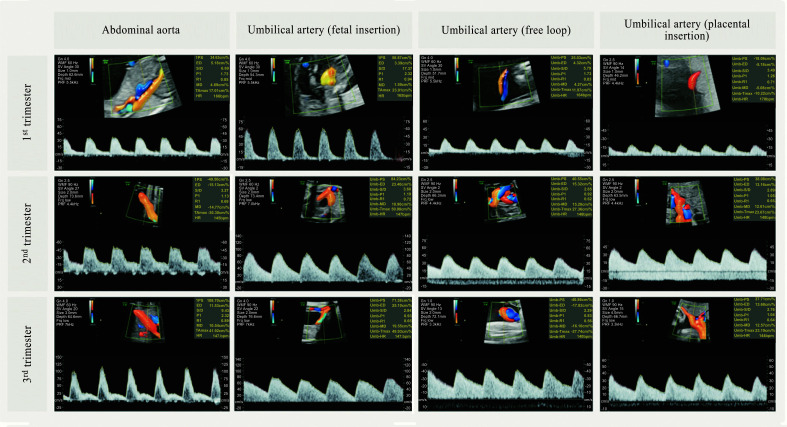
Aortoumbilical column Doppler assessment during pregnancy. From left to right, the evaluation point corresponds to: the fetal descending aorta; umbilical artery, intraabdominal portion; umbilical artery, free loop; umbilical artery, placental insertion. Throughout gestation, there is a noticeable decrease in PSV and an increase in EDFV, resulting in a decrease in impedance along the aortoumbilical column. These changes are more prominent in the first trimester when the trophoblastic invasion is not yet completed, leading to higher impedance indices on the fetal side. Additionally, an increase in impedance can be observed at the intraabdominal portion of the umbilical arteries at the bladder level. This may be attributable to increased resistance in the blood circulation of the lower limbs, maintaining an impedance differential between the low-resistance compartment (placental) and the fetal compartment, allowing blood to be equally distributed to both territories.
